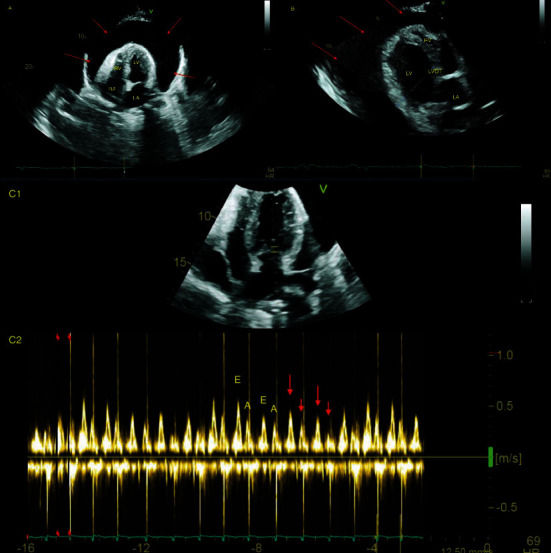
Transthoracic echocardiogram on day 0. Very large pericardial effusion with a swinging heart (red arrows in (a) and (b)), RV diastolic collapse, and marked mitral inflow respiratory variation (red arrows in (c)) consistent with significant tamponade. (a) Apical 4-chamber view; (b) parasternal long-axis view; (c) apical 4-chamber view showing the position of the Doppler pulse wave vector to obtain the mitral inflow waveform; (d) mitral inflow waveform.
